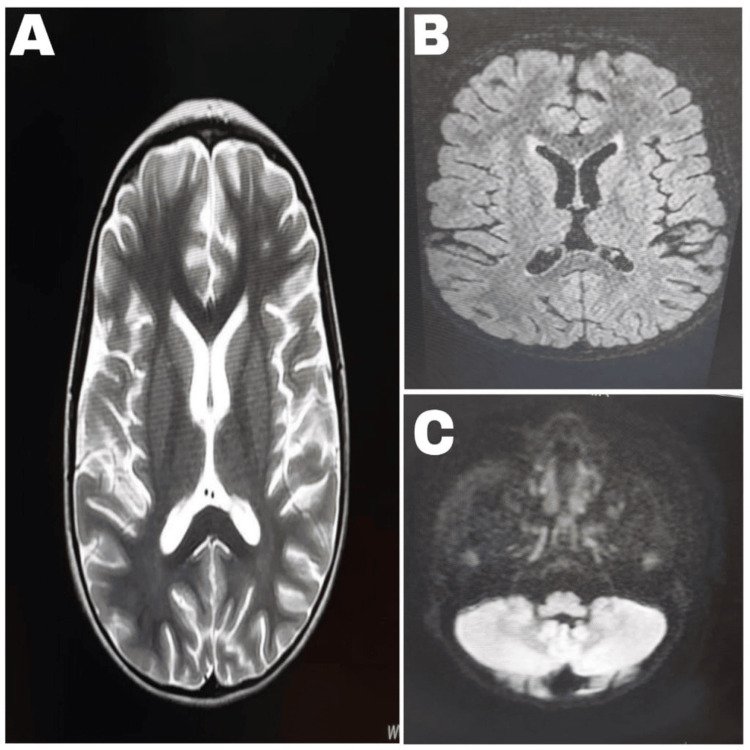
Follow-up brain magnetic resonance images showing complete lesion resolution. (A) Axial T2-weighted image showing disappearance of the splenial lesion. (B) Axial fluid-attenuated inversion recovery image showing complete resolution of the splenial abnormality. (C) Axial diffusion-weighted image showing complete resolution of the bilateral cerebellar lesions involving the dentate nuclei.
